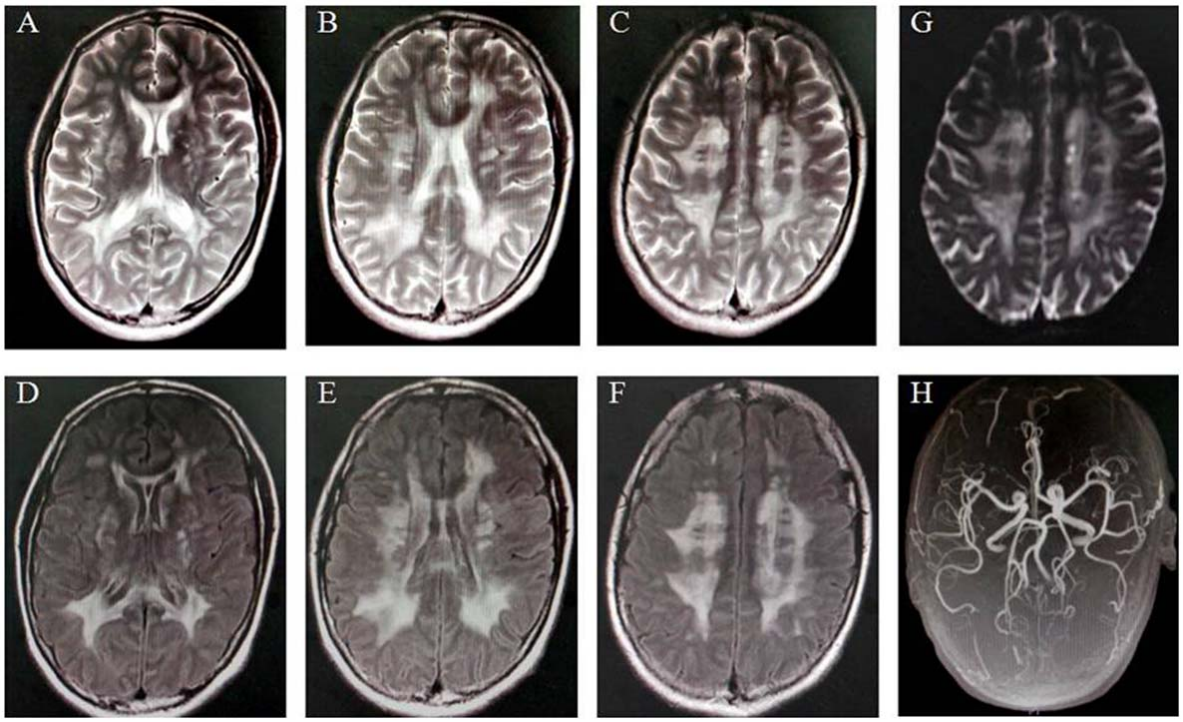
Brain MRI images of the proband showing characteristic diffuse leukoencephalopathy. T2-weighted MRI images (A–C) showed diffuse hyperintensities in the periventricular white matter with a symmetrical distribution, the centrum semiovale, and the basal ganglia. Fluid-attenuated inversion recovery (FLAIR) T2 sequences (D–F) revealed confluent white-matter lesions in multiple brain regions. Diffusion-weighted imaging (DWI) sequence (G) demonstrated acute ischemic stroke in the centrum semiovale. MR angiography (H) revealed no intracranial arterial stenosis or occlusion.
